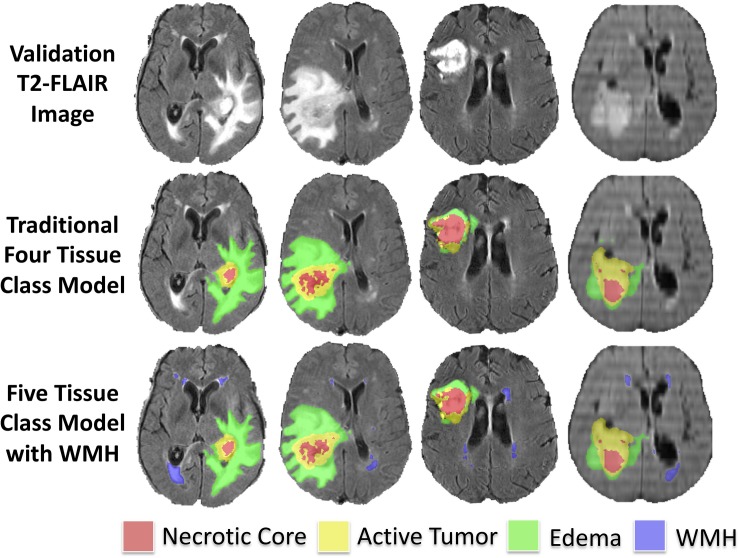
Example predicted segmentations in validation subjects with greater than 5 cm^3^ of WMH. Axial T2-FLAIR slices of four example validation cases are shown in the first row. The predicted tumor segmentations overlaid from the four-class model (background, AT, NCR, and ED) and the five-class model (background, AT, NCR, ED, and WMH) are shown in the second and third row, respectively.
